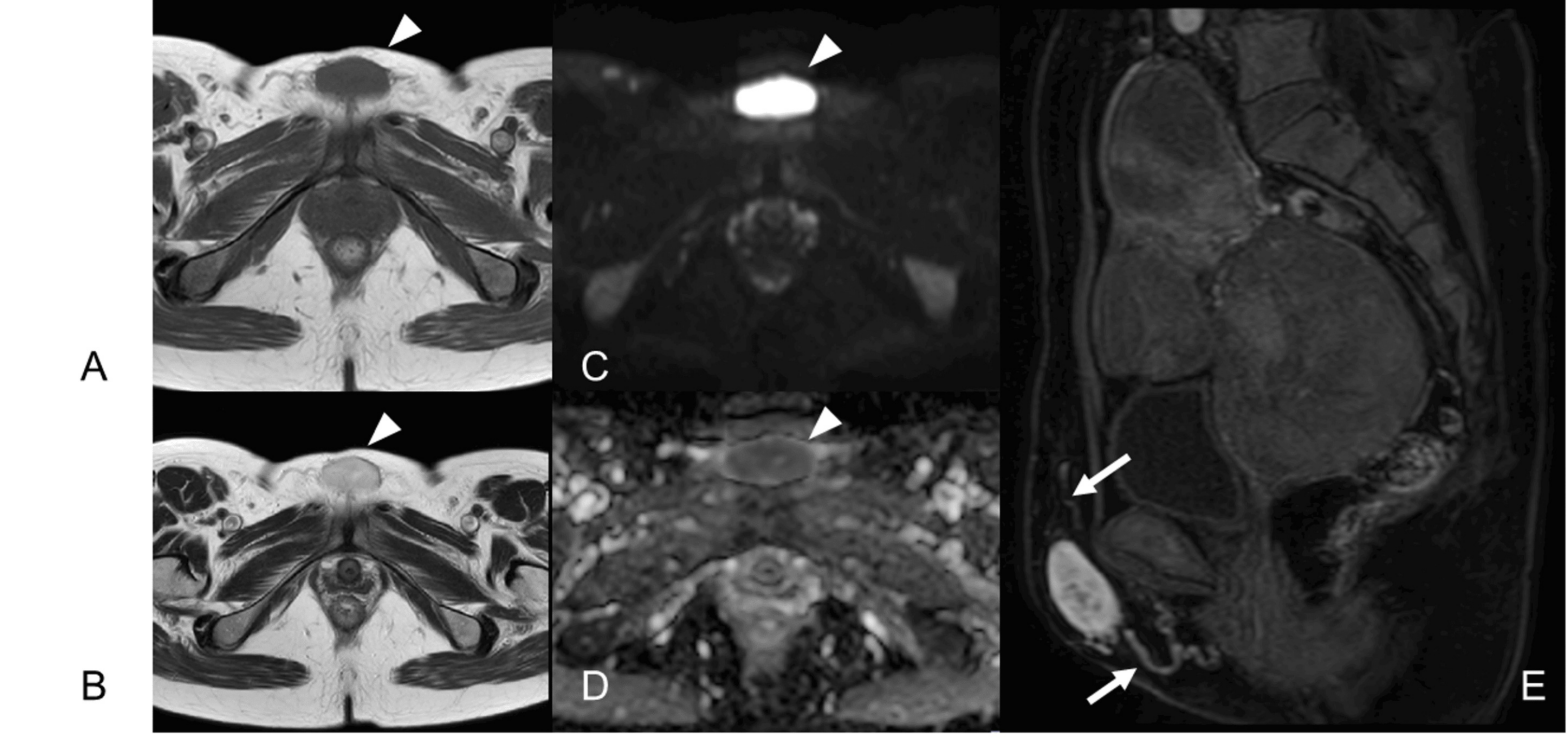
MRI of the pelvis (Case 1) A well-defined 3.9×3.9×2.0 cm tumor (arrowheads) is present within the subcutaneous fat tissue, showing iso-intensity on T1-weighted image (A: axial view) and high-intensity with a low-intensity capsule on T2-weighted image (B: axial view), high-intensity on DWI (C: axial view), and low-intensity on ADC (D: axial view). The tumor showed almost homogenous enhancement with gadolinium on T1-weighted fat suppression, and tortuous vascular structures (arrows) were proliferating around the tumor (E: sagittal view). DWI: diffusion-weighted imaging
